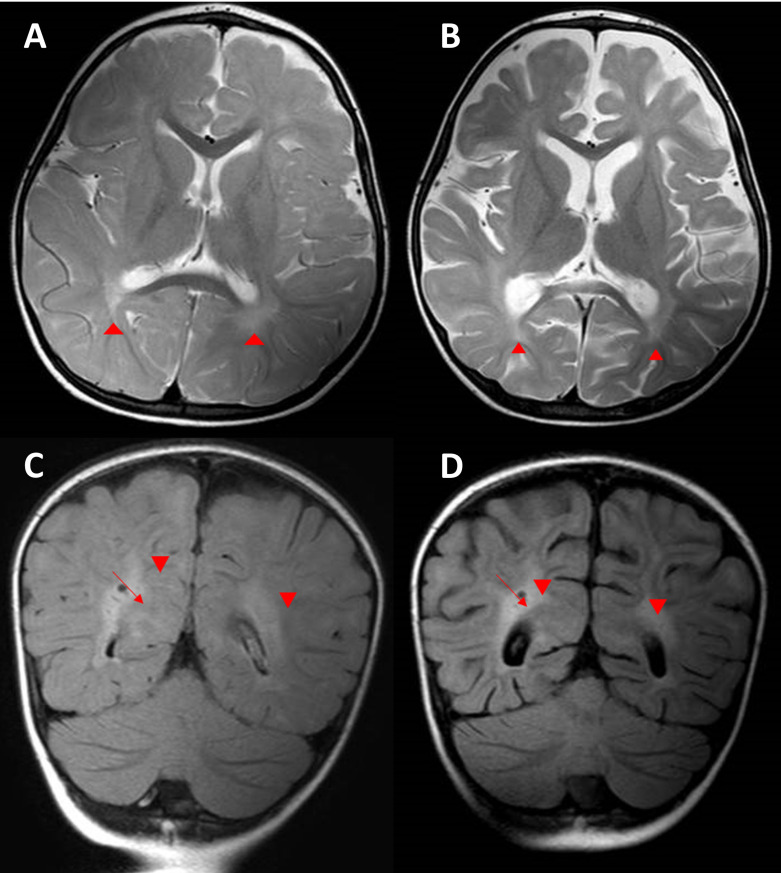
Proband axial brain MRI scans. (A) Axial T2 brain MRI showing delayed myelination for the patient’s age with diffuse abnormal high T2 signal intensity seen in the periventricular deep white matter, more prominent in both peri-trigonal regions (arrow heads). There is also prominence of the subarachnoid spaces in both frontal regions. (B) Follow-up axial T2 brain MRI demonstrating progressive brain atrophy with unchanged delayed myelination and diffuse abnormally high T2 signal intensity seen in the periventricular deep white matter, more prominent in both peri-trigonal regions (arrowheads). (C) Coronal FLAIR brain MRI image demonstrating diffuse abnormally high signal intensity in the periventricular deep white matter, more prominent in both peri-trigonal regions (arrow heads) with prominent VR spaces (arrow). (D) Follow-up coronal FLAIR brain MRI demonstrating the progressive brain volume loss with unchanged diffuse abnormally high signal intensity in the periventricular deep white matter, more prominent in both peri-trigonal regions (arrowheads) with prominent VR spaces (arrow).
